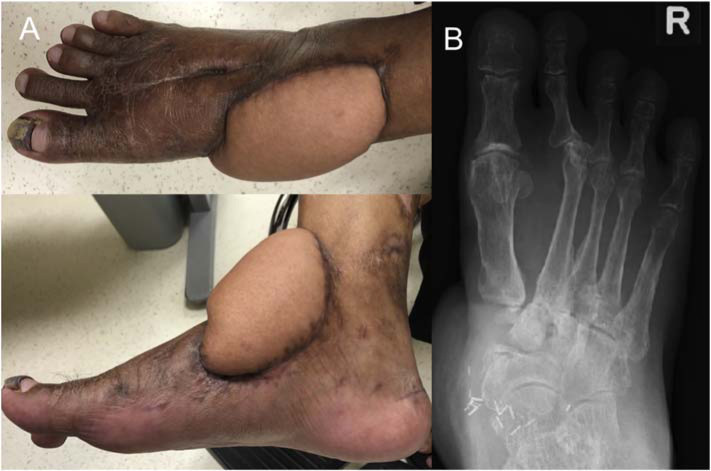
A: Clinical photos of the patient's flap 10 months post-injury. B: Radiograph at latest follow-up.
